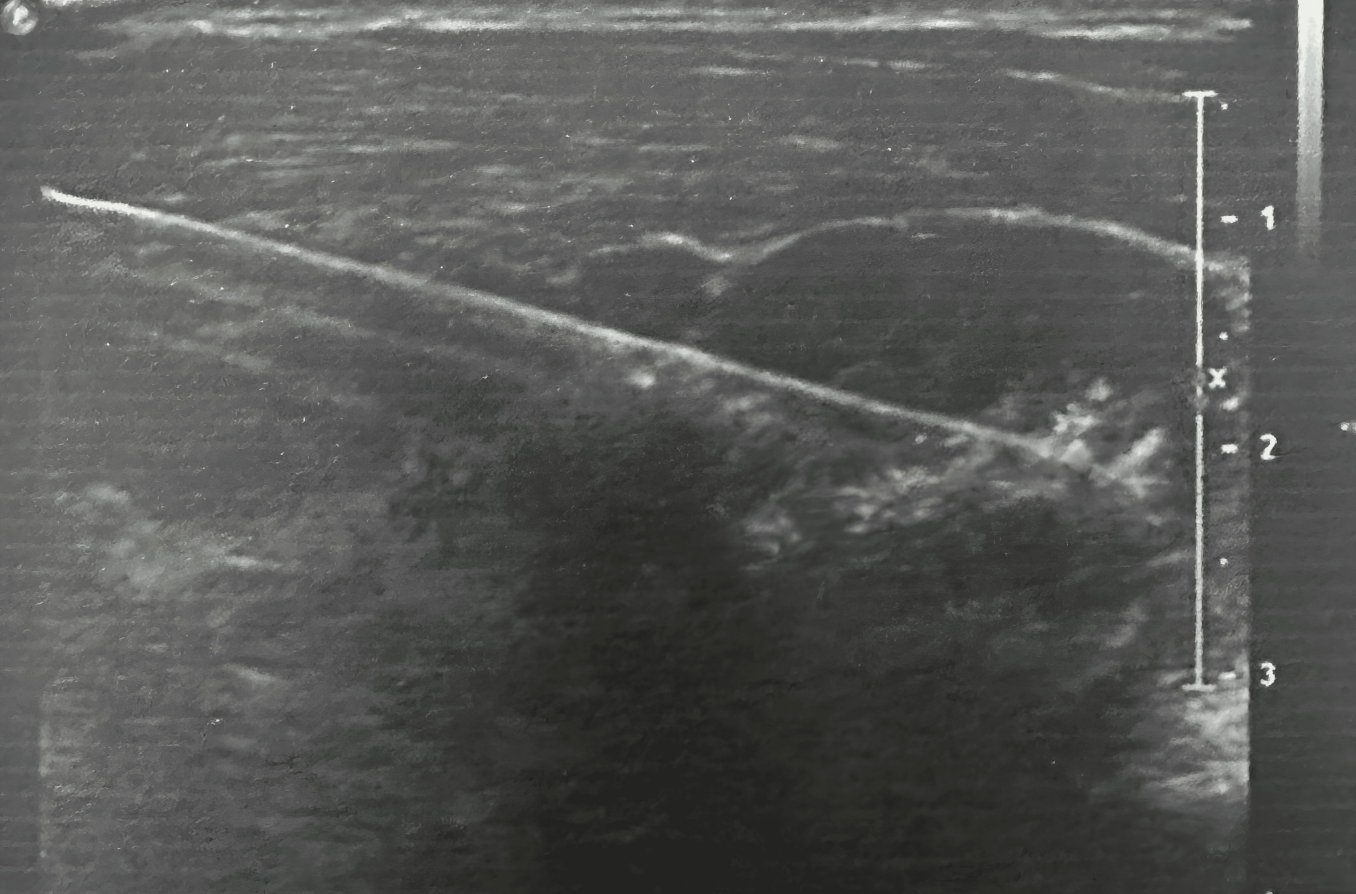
Ultrasound-guided drainage of a breast abscess
